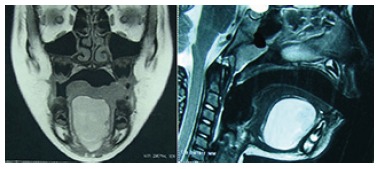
Magnetic resonance imaging showing oval cystic formation and rounded images
